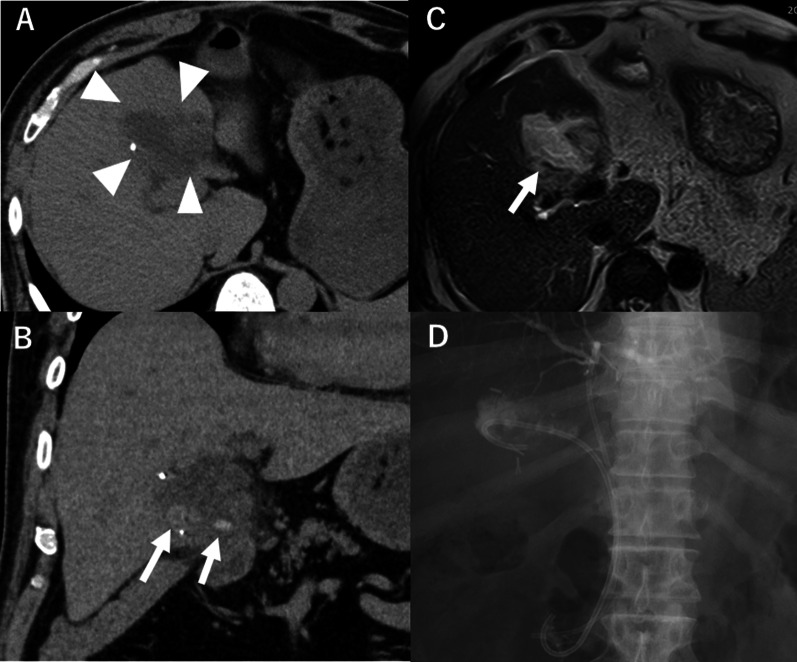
Case 1. a Plain abdominal computed tomography. 38 × 36 × 35 mm remnant gallbladder was found (arrowhead), b Gallstone was found in the remnant gallbladder (arrow). The fat tissue concentration around the remnant gallbladder was elevated. c T2-weighted MR imaging identified the remnant gallbladder with fluid collection around it (arrow). d EBS and EGBD tube were placed in the common bile duct and the remnant gallbladder by ERCP, respectively
